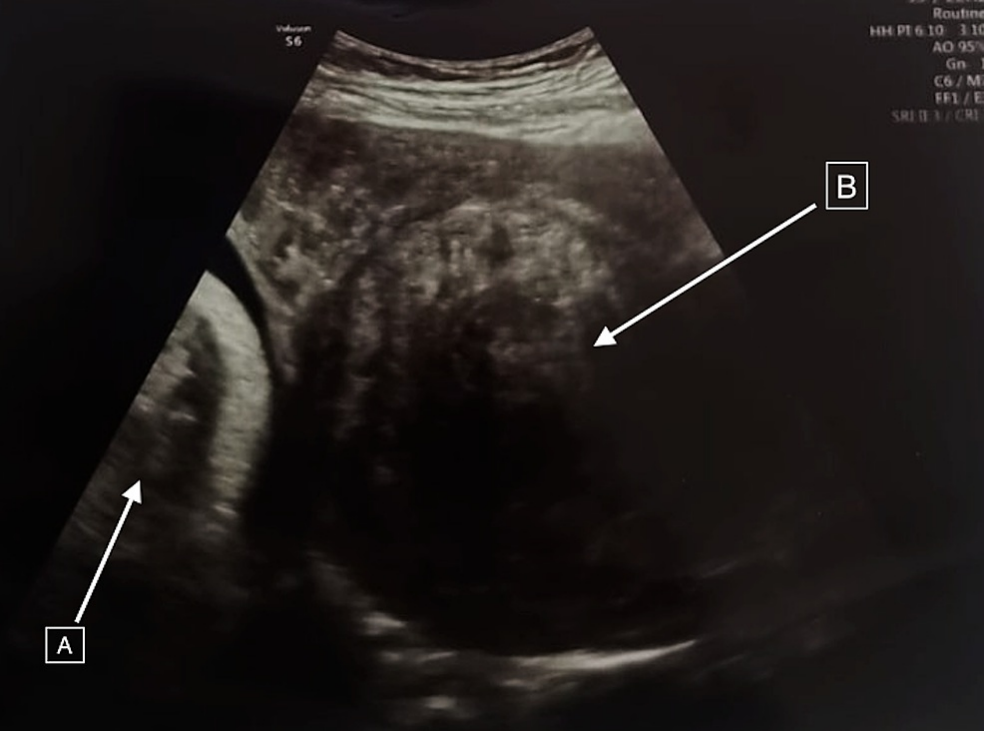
An abdominal ultrasound showing: A. The fetus’s head. B. The obstructing fibroid.
